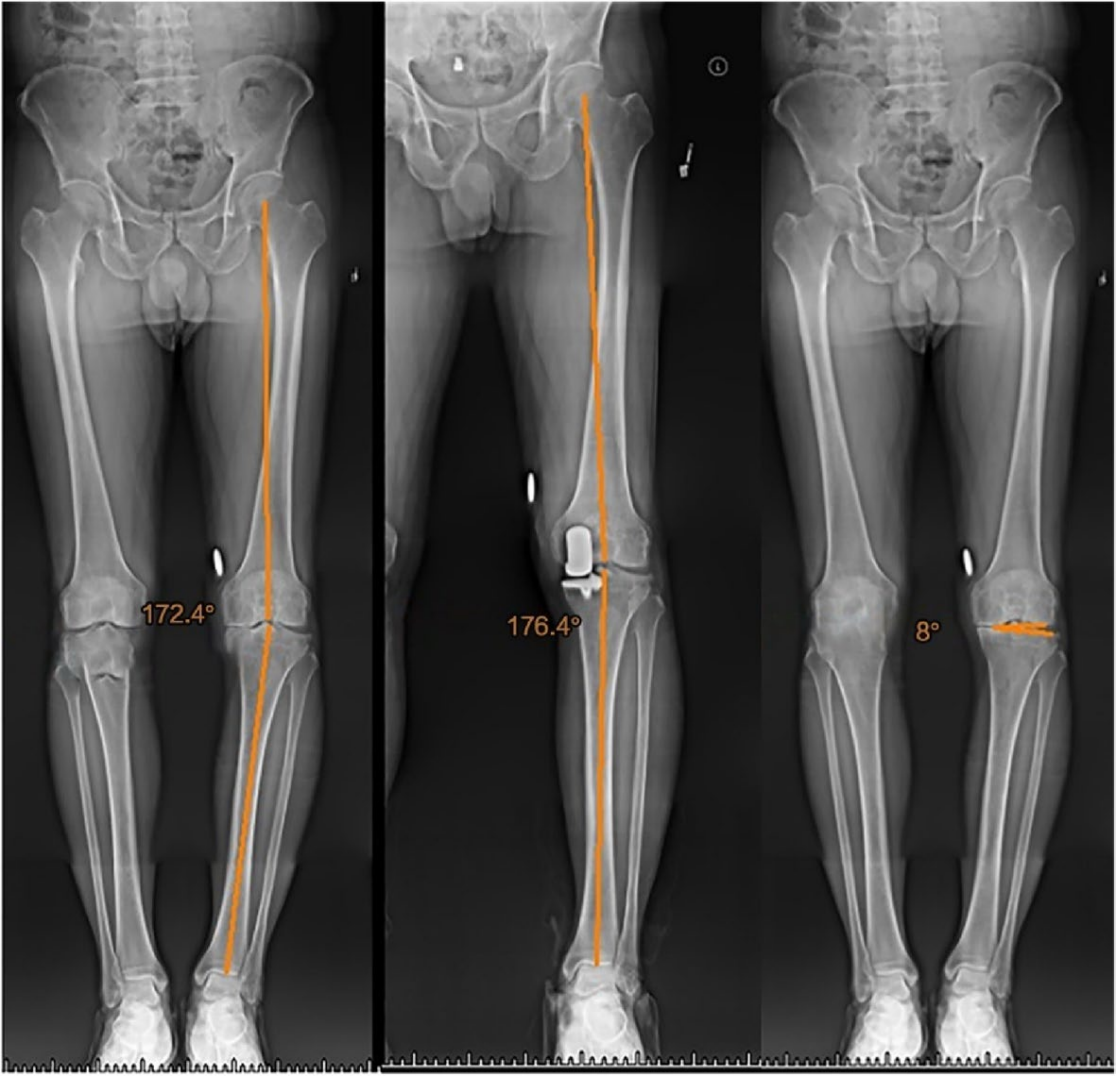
Preoperative and postoperative hip-knee-ankle angles and preoperative joint line convergence angle was measured in full-length radiograph
